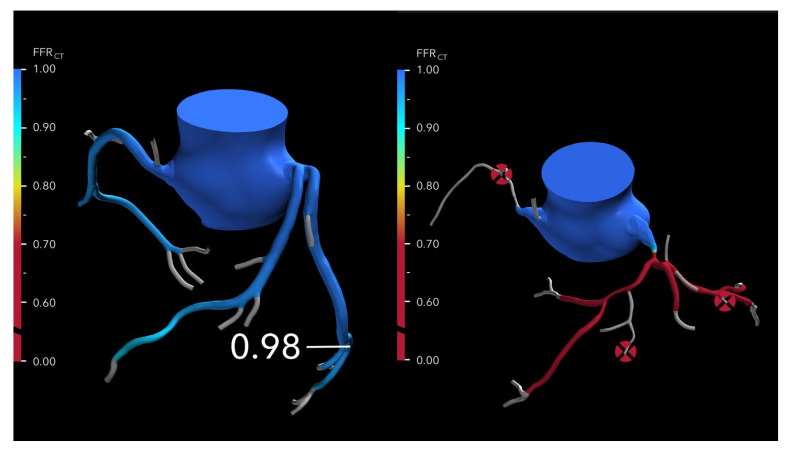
FFR-CT results are displayed using 3D-modeled representations of coronary arteries. (Left): A value above 0.80 is considered normal and is shown in blue. Borderline values ranging from 0.76 to 0.80 are depicted in orange and yellow. (Right): Values of 0.75 or below are classified as abnormal and marked in red. Areas of complete occlusion are indicated with an “X” symbol.
